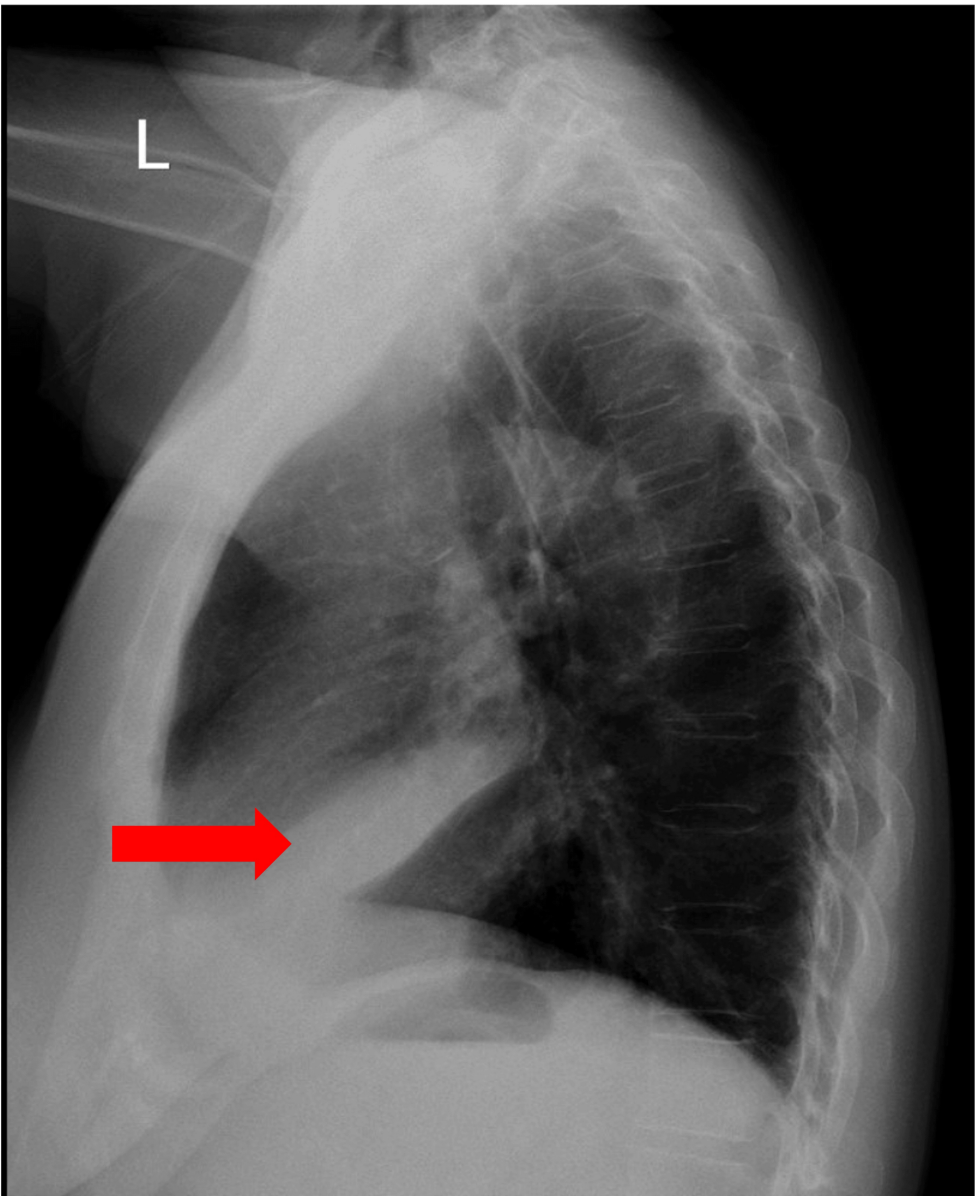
Lateral view of the chest X-ray showing consolidative opacity in the right middle lobe (red arrow), suggestive of infiltrates or atelectasis.
